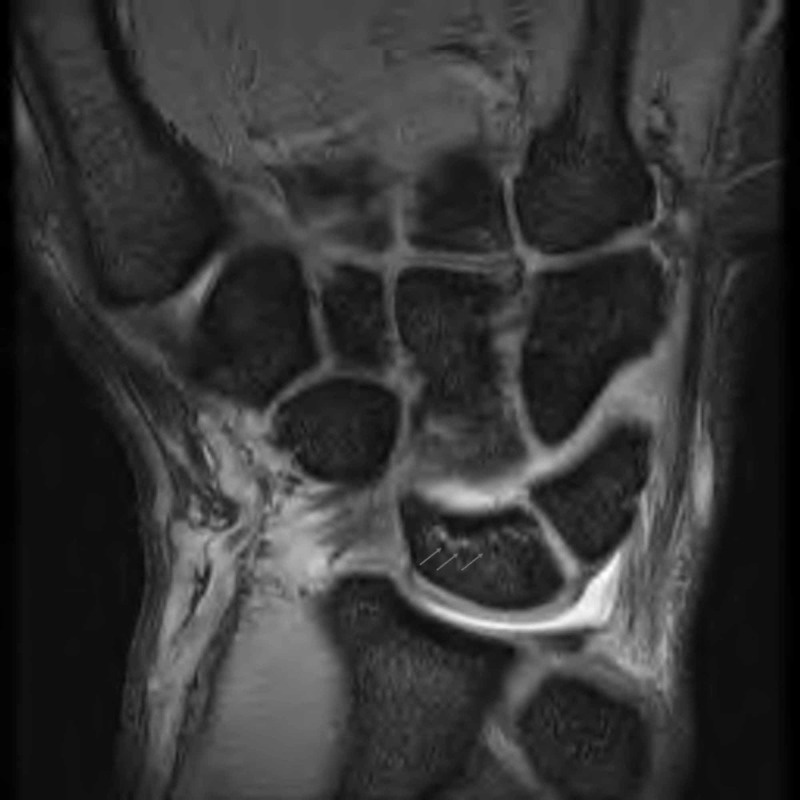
Coronal T2-weighted MR image showed mild increase in the signal intensity of the lunate bone.
